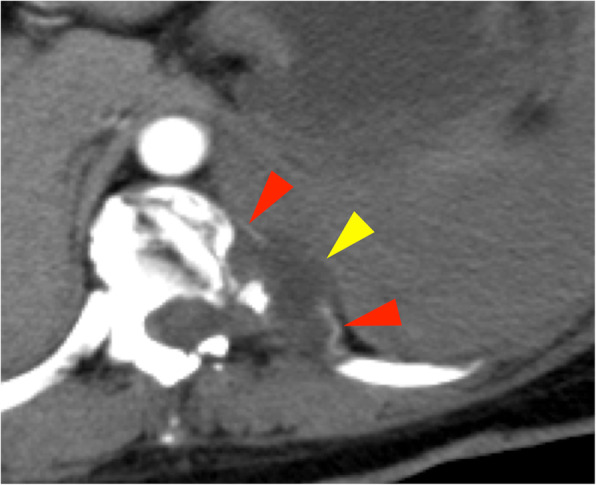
Chest computed tomography showed a massive hemothorax and an irregularly shaped left 10th intercostal artery (red arrow). Intrathoracic dural ectasia was observed close to the artery (yellow arrow)
